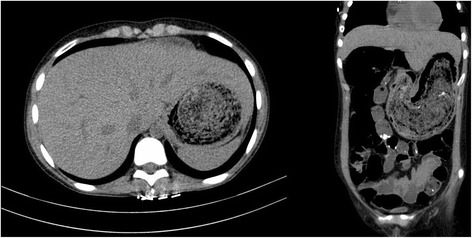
CT, Coronal and sagittal view: huge, well defined, multi-layered, heterogeneous, solid appearing, mass filling the gastric fundus and antrum and extending into duodenum. Since trichobezoar has an extension to the small bowel, it is classified as Rapunzel syndrome
